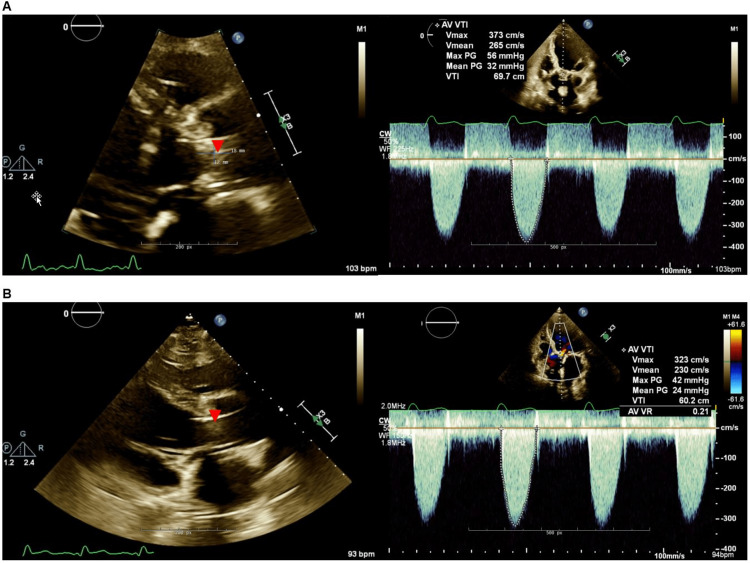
(A) Echocardiogram demonstrating a bioprosthetic aortic valve thrombus (red arrowhead) measuring 12 mm x 18 mm in parasternal long axis view with Doppler mode demonstrating a peak systolic velocity of 3.7 meters/second (m/s) and a mean gradient of 32 mmHg. (B) Echocardiogram after treatment showing improvement in the size of valvular thrombus (red arrowhead) associated with a decrease in peak systolic velocity to 3.2 m/s and mean gradient to 24 mmHg
